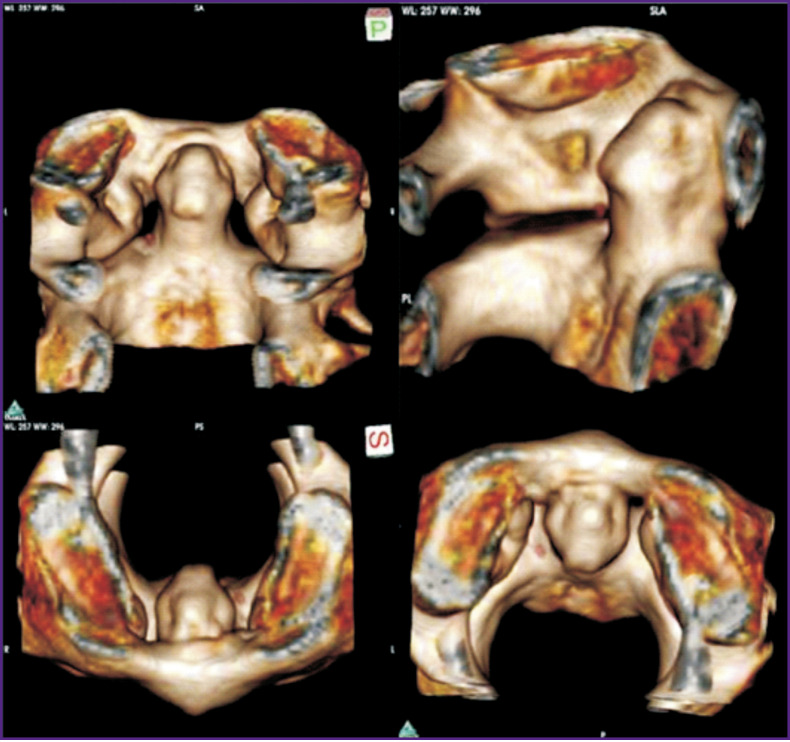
3D reconstruction of a CT image fragment of median atlantoaxial joint for a 69-year old man
